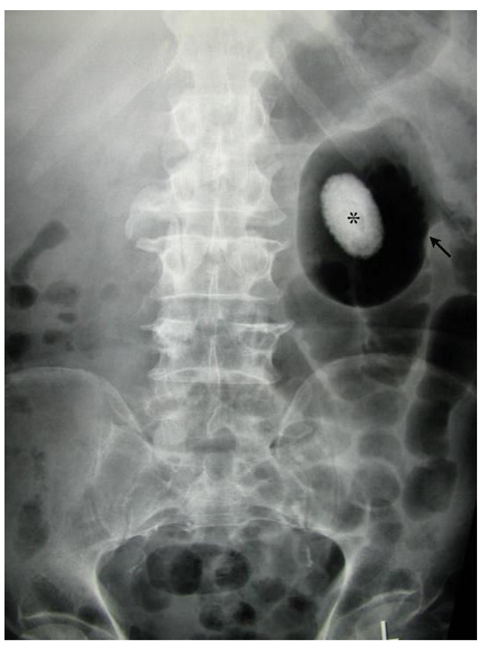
Plain abdominal radiograph showing oval radiopacity (*) surrounded by a radiolucent rim (arrow) at the region of the left kidney.
